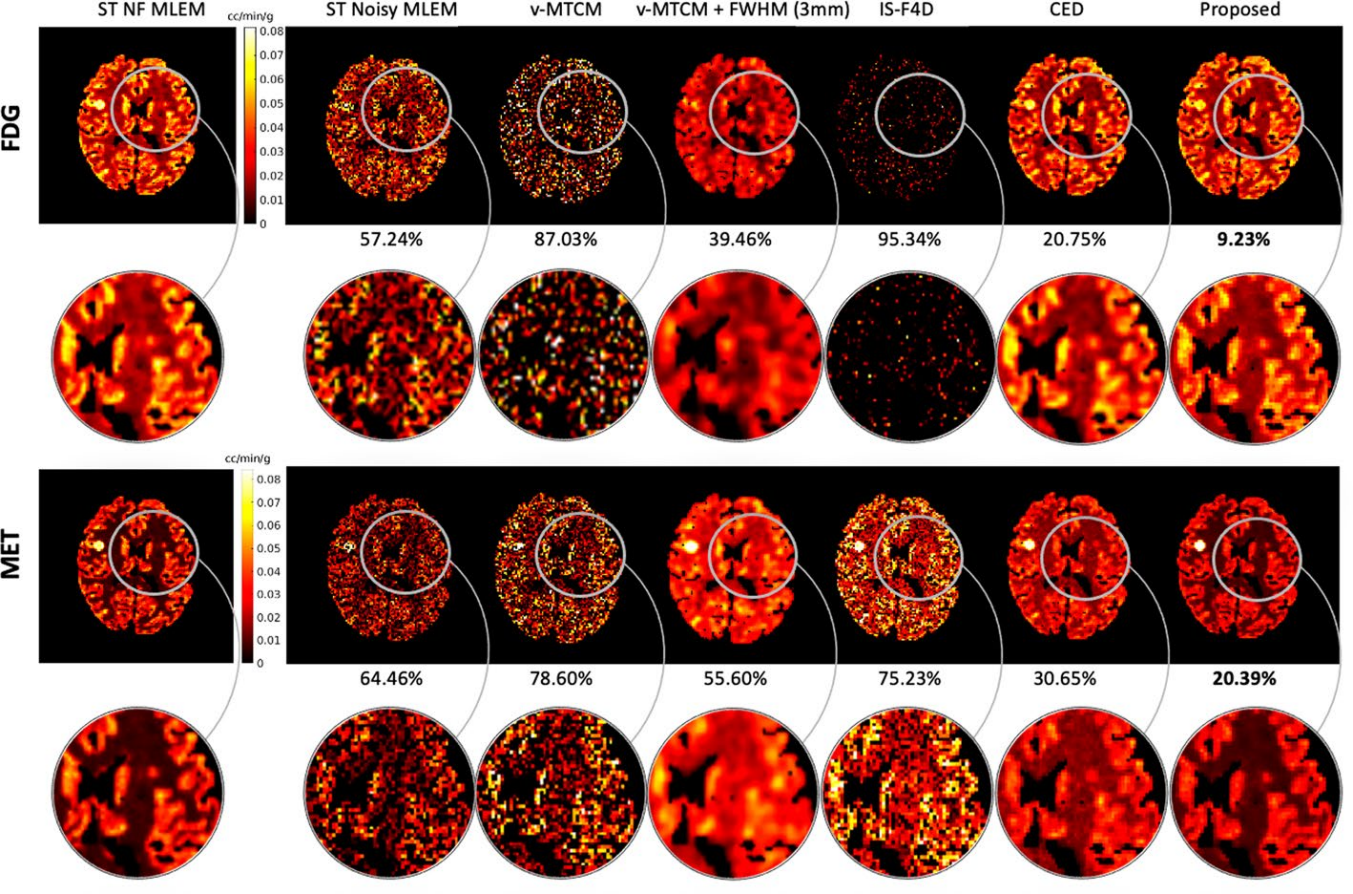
A test example of the parametric maps $$K_i$$ estimated from the separated single-tracer activity images for FDG and MET, respectively. NRMSE values of each estimated $$K_i$$ image were shown at the bottom. For plotting only, we limit the colour scale of the figures to fall within the same range as the reference images shown in the first column
